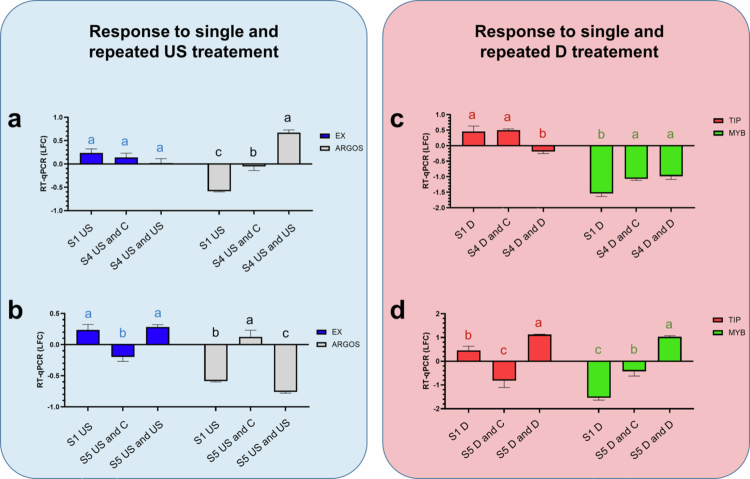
Effect of single and repeated exposure to stress stimuli on expression intensities of target genes at two time points. Differential expression of target genes (logarithmic fold change (LFC) values) at 2 d (S1), 7 d (S4) and 12 d (S5) by different treatments (ultrasound and drought stress), as assessed by RT-qPCR. All LFC values were in comparison with the absolute control group (C at S1; C and C at S4, S5). Abbreviations: S1 US – value measured 2 d after ultrasound treatment (S1); S4 US and C – value measured 7 d (S4) after single ultrasound treatment; S5 US and C – value measured 12 d (S5) after single ultrasound treatment; S4 US and US – value measured 7 d (S4) after initial application of ultrasound stress and 2 d after repeated ultrasound stress; S5 US and US – value measured 12 d (S5) after initial application of ultrasound stress and 2 d after repeated ultrasound stress; S1 D – value measured 2 d (S1) after applying single drought stress; S4 D and C – value measured 7 d (S4) after applying single drought stress; S5 D and C – value measured 12 d (S5) after applying single drought stress; S4 D and D – value measured 7 d (S4) after initial application of drought stress and 2 d after repeated drought stress; S5 D and D – value measured 12 d (S5) after initial application of drought stress and 2 d after repeated drought stress. Error bars represent the standard deviation of the mean. Different lower-case letters above the error bars indicate significant differences at the 0.05 level (two-way ANOVA and post hoc Tukey’s B test results).
